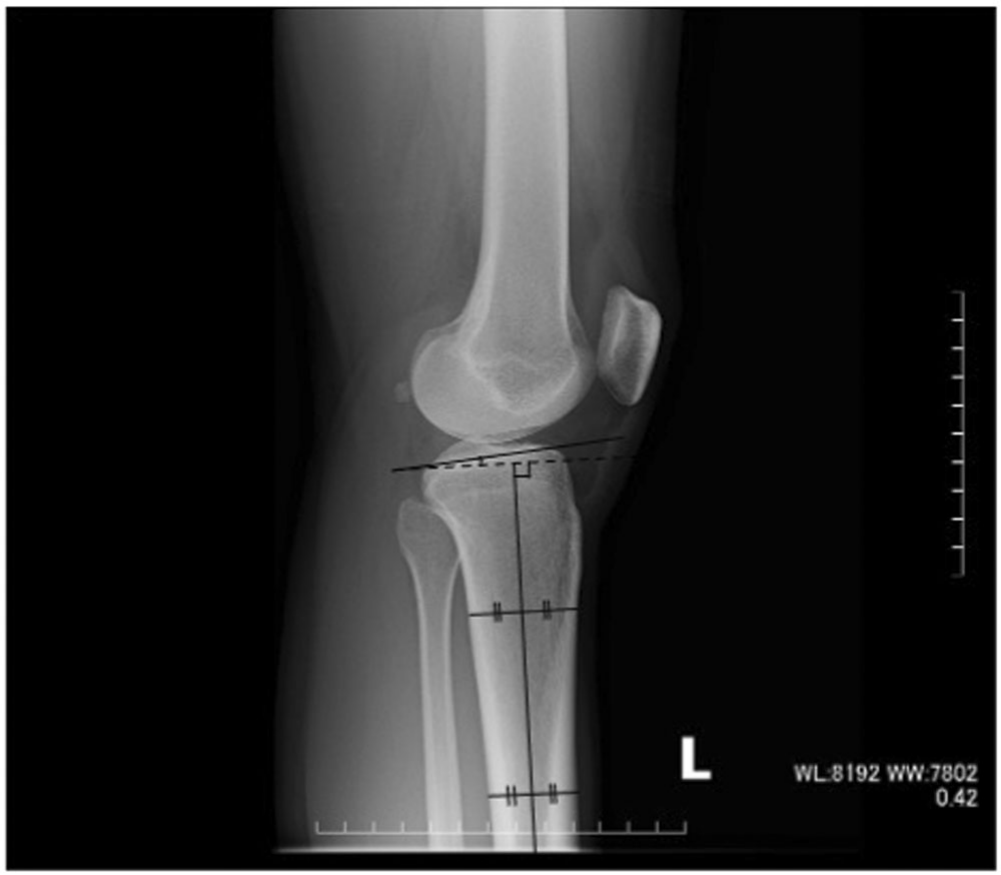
Lateral radiograph of the knee in the sagittal plane. Measurement of the posterior tibial slope angle: The posterior tibial slope angle was determined as the angle between the line perpendicular to the tibial mechanical axis and the line connecting the most proximal anterior and posterior points of the medial tibial plateau [25].
